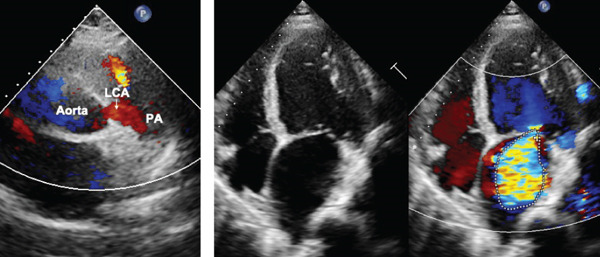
Echocardiogram showed ALCAPA (left) and mitral regurgitation (right). Left: The left coronary artery (LCA) originates from the pulmonary artery (PA). Right: The left atrium was markedly enlarged and severe mitral valve regurgitation was shown.
